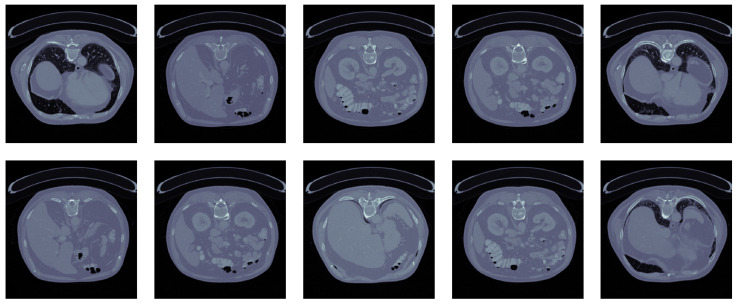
Representative samples from the CT-based liver tumor dataset used in this study.
